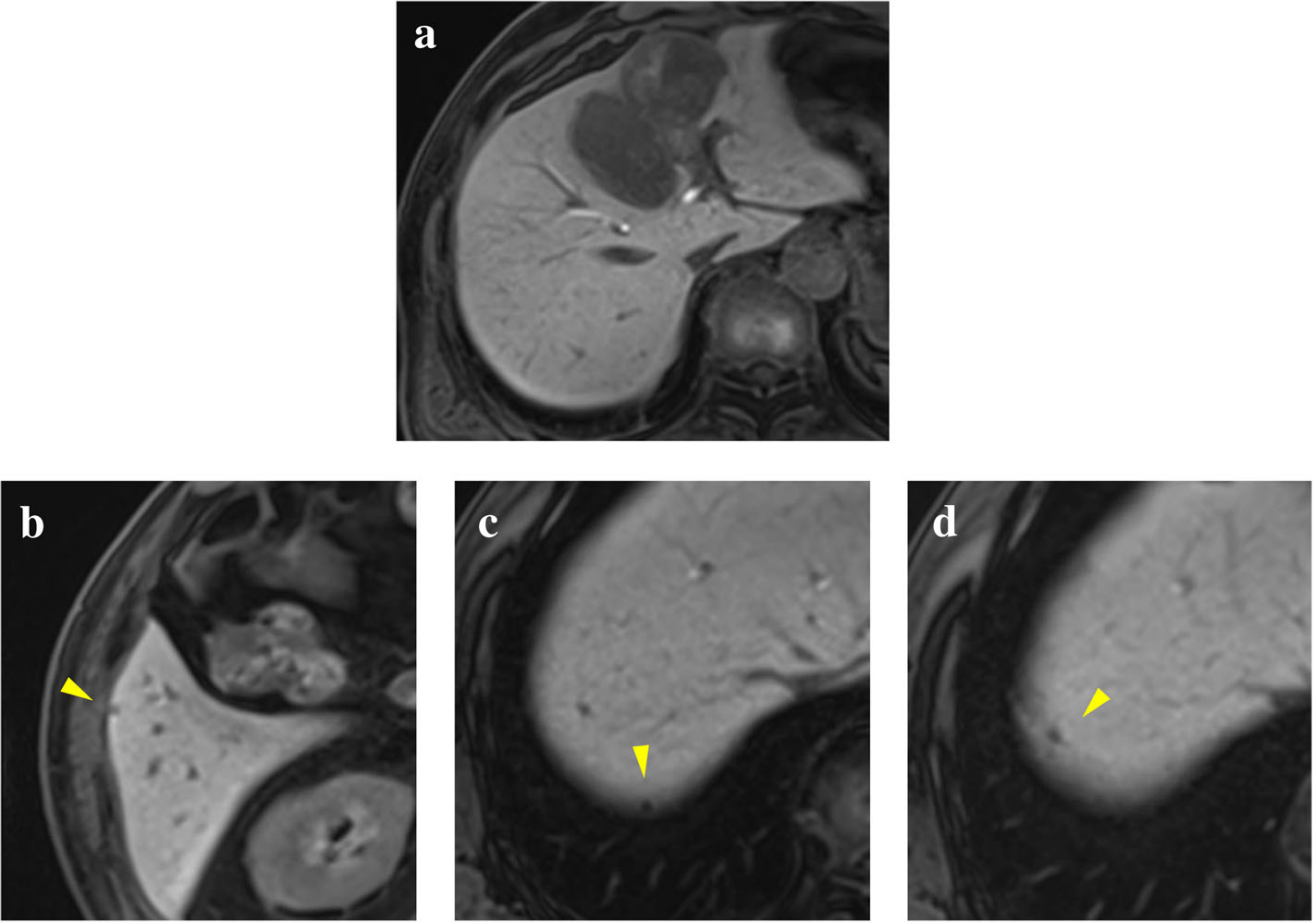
Gadolinium-enhanced magnetic resonance imaging findings. In addition to main tumor in S3+4 (a), small nodules that represented enhancement defect in the hepatocyte phase were detected in S6 (2b), S7 (2c), and S8 (2d)
